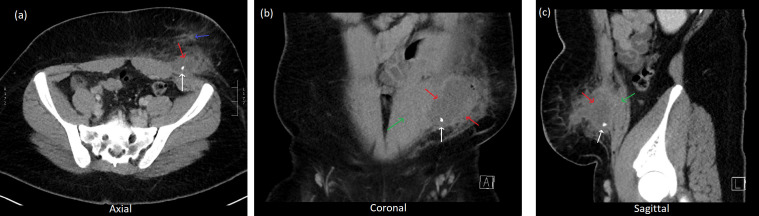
CT 11 months post op. (a) Axial (b) Coronal
